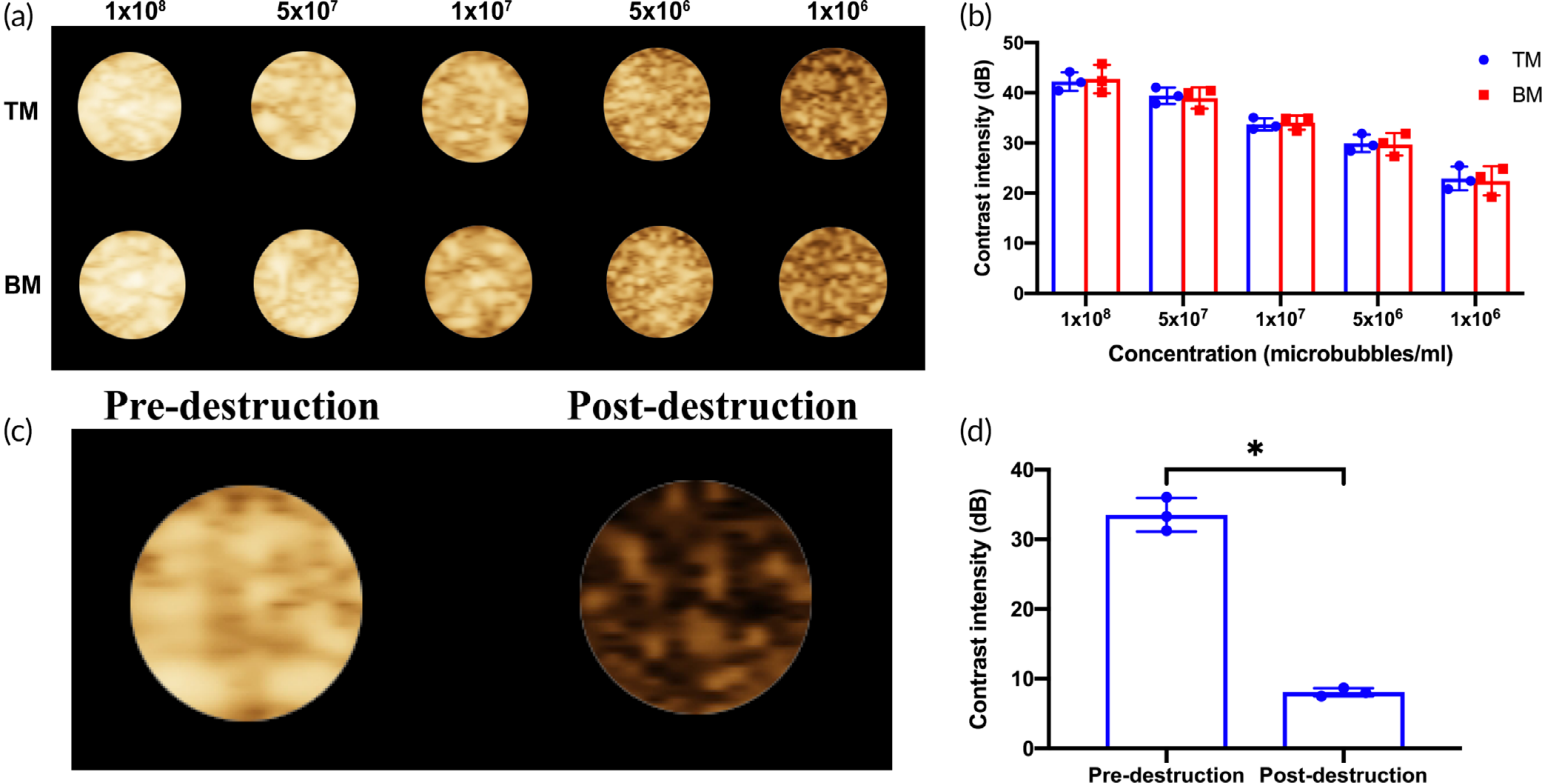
In vitro imaging of TM. (a) Ultrasound images of microbubbles at different concentrations in vitro. (b) Quantification of contrast intensity of microbubbles in vitro (*n* = 3 per group). (c) Ultrasound images of TM before and after destruction. (d) Quantification of contrast intensity of TM before and after destruction (*n* = 3 per group). Data in the graphs represent the mean ± SD, and *p* values were determined using *t* test. p* < 0.05. BM, blank microbubbles; TM, targeted microbubbles.
